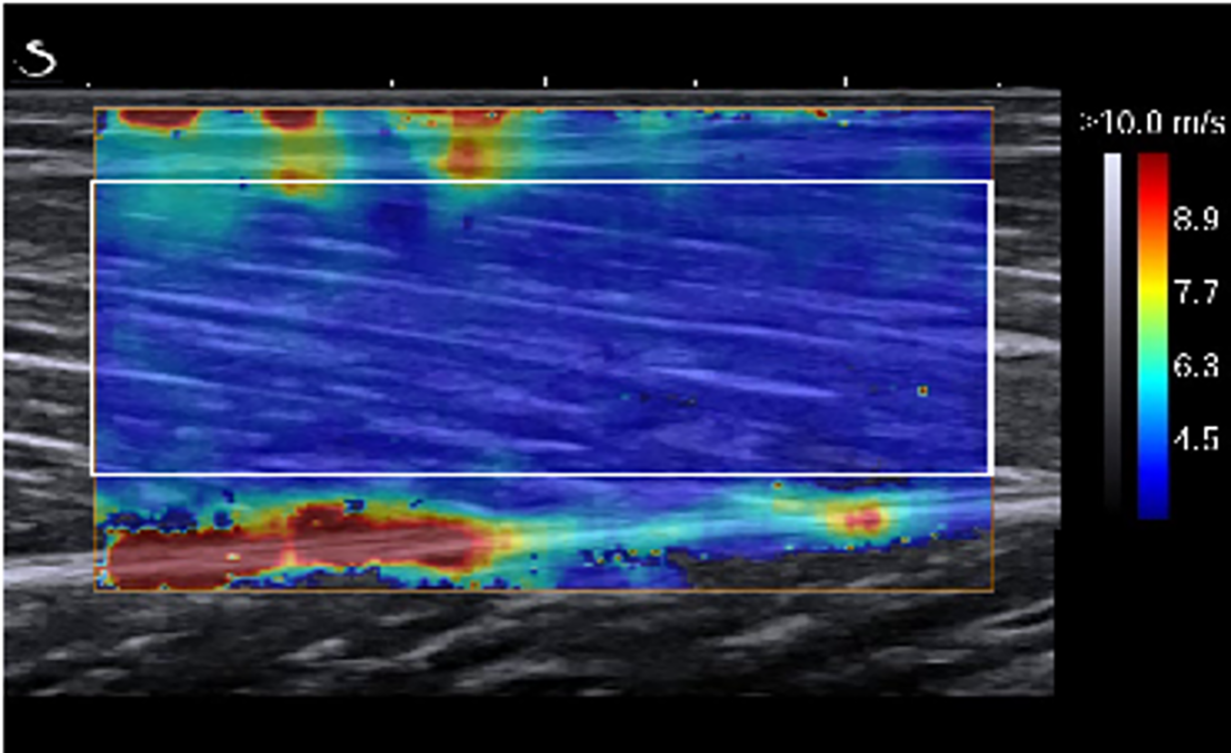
Shear wave speed measurement from the shear-wave ultrasound elastography image at the neutral ankle position. The rectangle represents the region of interest (ROI) between the superficial and deep aponeuroses, and the colored region represents the shear elasticity map with the scale to the right of the figure.
